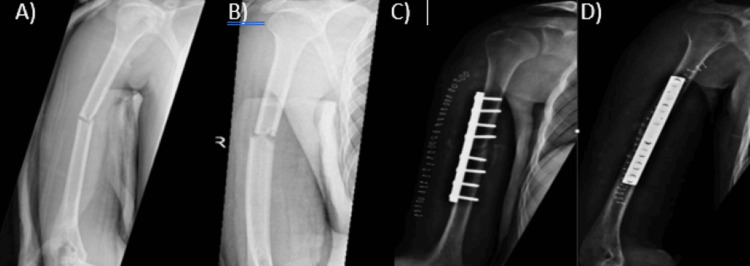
Anteroposterior (AP) and lateral radiographs of the right humerus shaft fracture fixed with an anterolateral plate. (A) Preoperative anteroposterior (AP) view, (B) preoperative lateral view, (C) postoperative anterolateral plate fixation AP view, (D) postoperative anterolateral plate fixation lateral view
